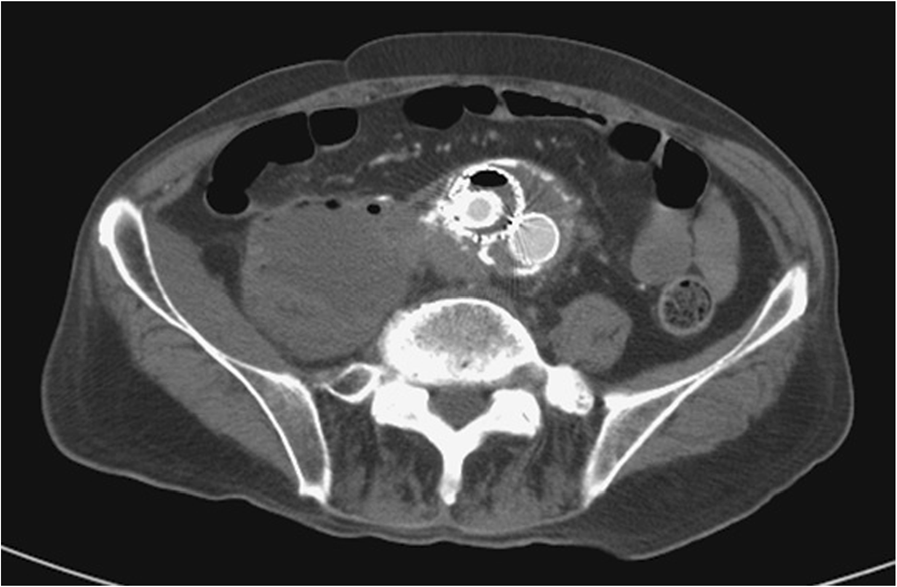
Abdominal CT depicted air within the endograft and emphysematous abscess of the right IPM
